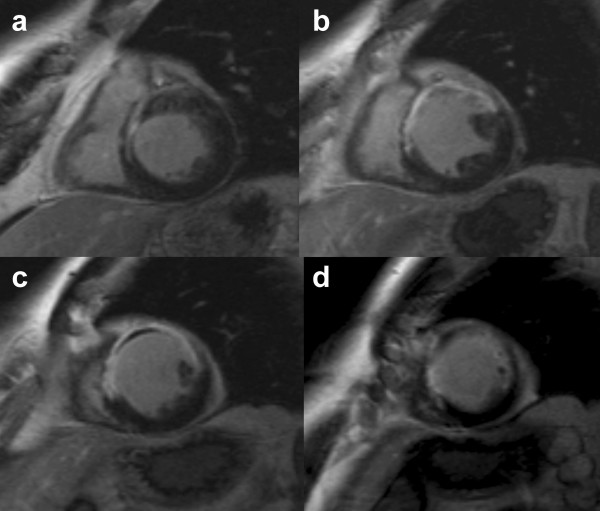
Short axis basal (a), mid-ventricular (b, c) and distal (d) segmented Inversion Recovery gradient echo MR images 10 minutes after bolus injection of Gadolinium-DTPA, showing a large region of myocardial damage (hyperenhancement surrounding region of persistent hypoenhancement) in the antero-septal territory (same patient than Figure 1).
